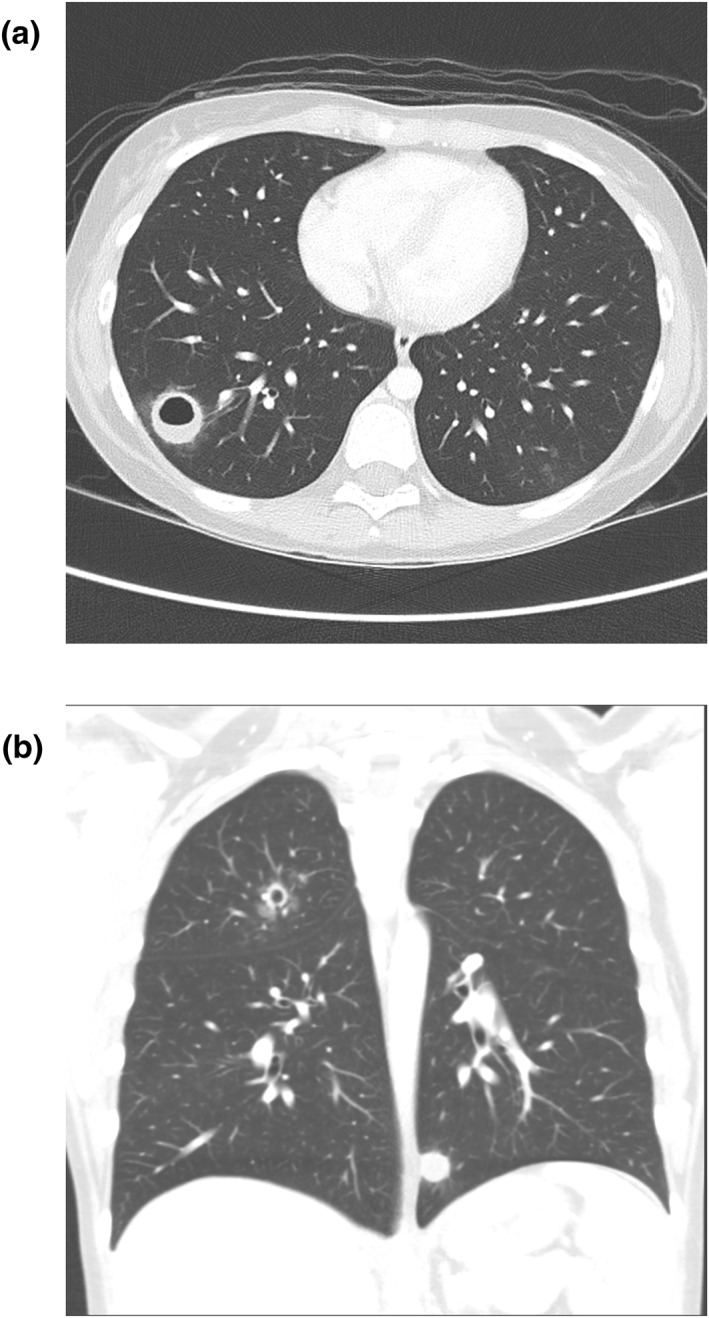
(a, b) Axial and coronal CT Chest with contrast showing right upper lobe cavitary lesion and left upper lobe nodule both with associated surrounding ground glass changes.
